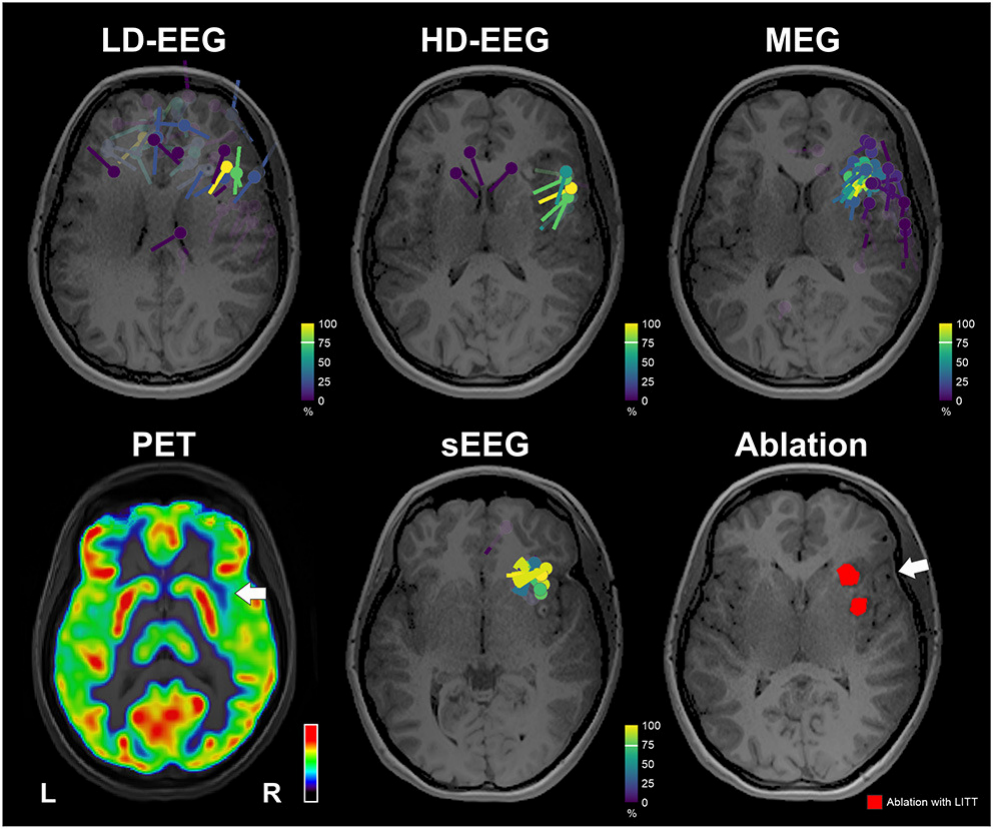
Neuroimaging findings with respect to ablation. Upper: Dipoles clustering overlaid on patient's MRI for the conv-EEG, HD-EEG, and MEG. Dipoles are color-coded based on clusterness [dipoles with high clusterness (have several other dipoles in its vicinity) are depicted with yellow; dipoles with low clusterness (few or no other dipoles in its vicinity) are depicted with magenta]. Lower: FDG-PET image shows a small region of hypometabolism at the anterior right superior temporal gyrus, indicated by white arrow. Dipoles after clustering for iEEG. Ablation volume (red) overlaid on patient's MRI. White arrow indicates the location of a possible focus of gliosis identified on patient's MRI. MRI and PET are displayed on neurological orientation (left to left).
